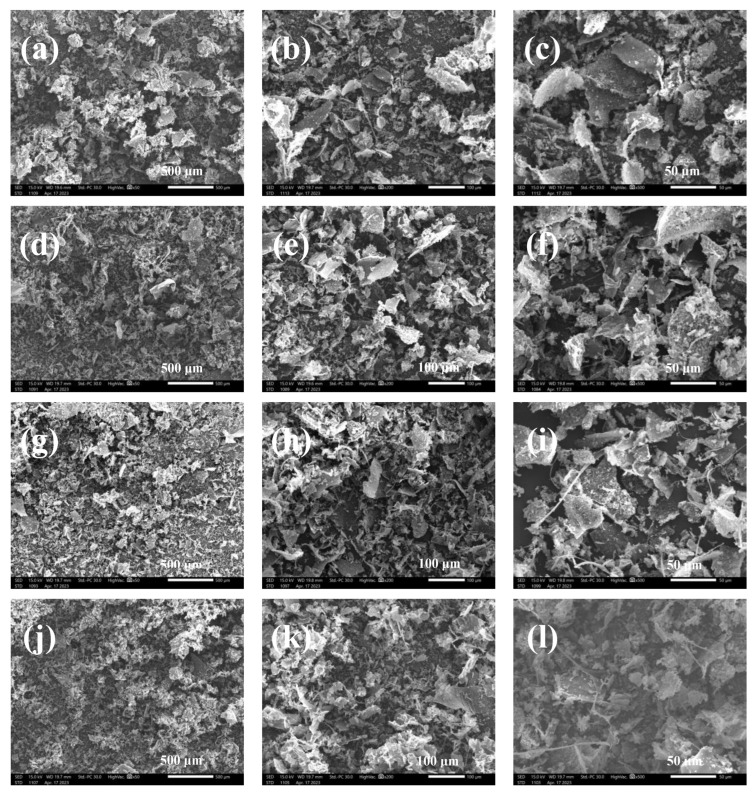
(a–c) SEM with different magnified images of LESP without ultrasonication treatments and (d–l) SEM with LESP obtained under different ultrasonication conditions.
